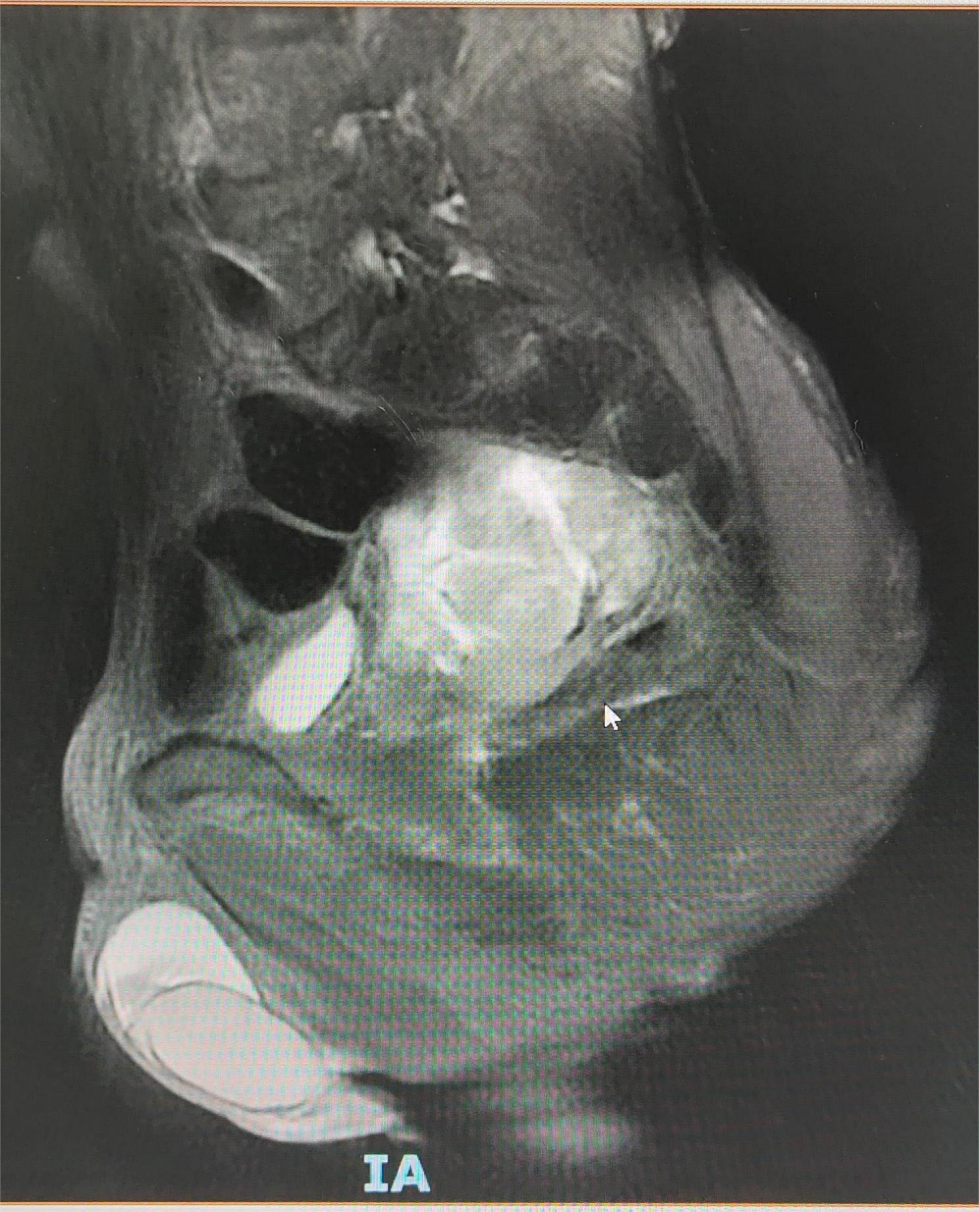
Pelvic MRI showing a blood-rich tumor-like lesion on the left side of the pelvis, poorly demarcated from the left seminal vesicle gland and prostate.
